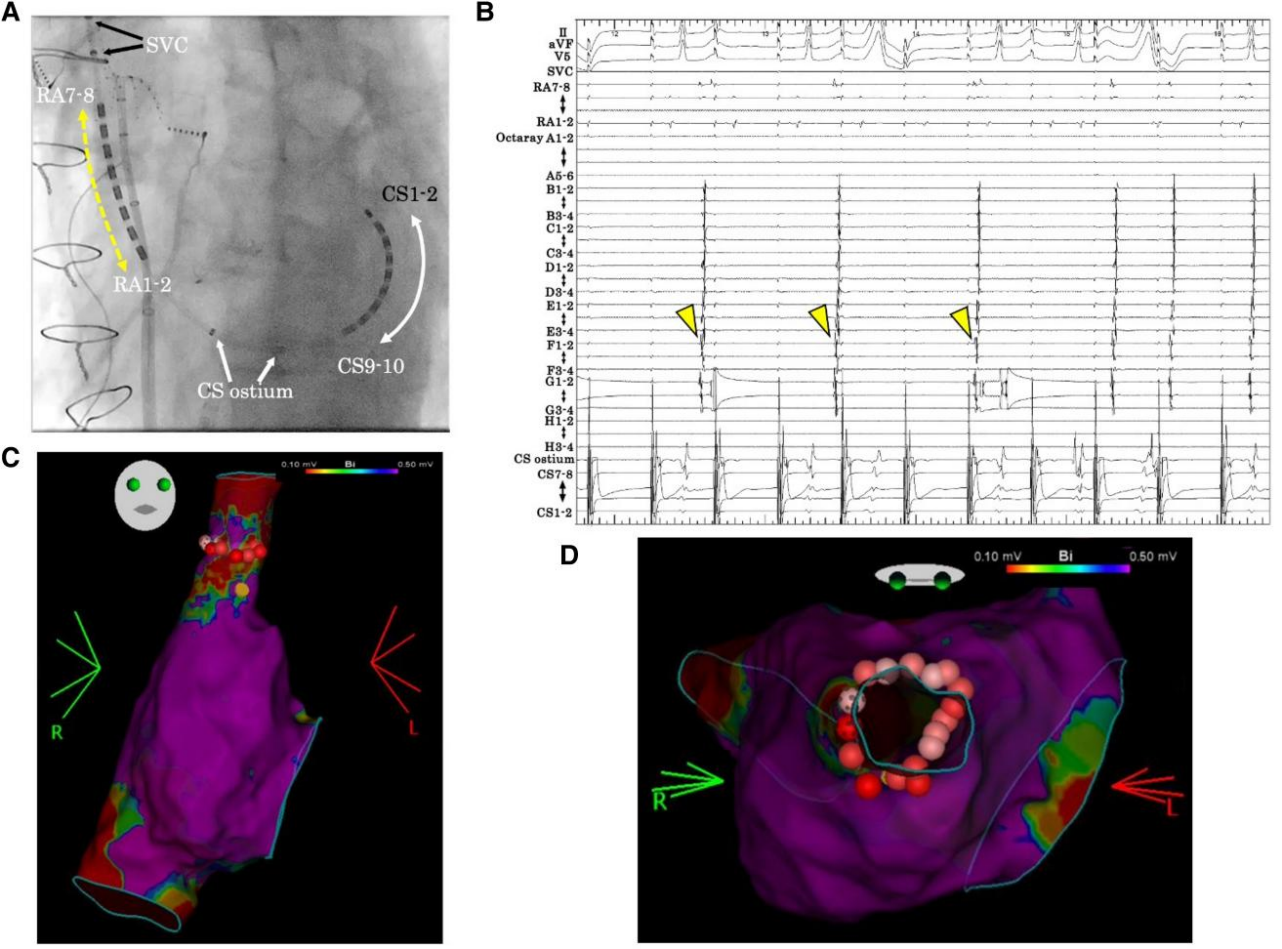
(*A*) Fluoroscopic images showing the position of an A BeeAT catheter in the superior vena cava (black arrow), right atrium (yellow arrow), and coronary sinus (white arrow). The Octaray catheter is positioned in the superior vena cava. (*B*) Intracardiac electrograms after adenosine triphosphate administration. During constant pacing from coronary sinus 5–6, ectopic activity originating from the superior vena cava was observed (yellow arrow). (*C* and *D*) Right atrial activation map after pulmonary vein isolation, merged with left atrial computed tomography image, demonstrating dormant conduction. Superior vena cava isolation was performed using thermal ablation (red tag). High-power, short-duration applications (dotted red tags) were delivered selectively to avoid phrenic nerve injury. Circumferential lesions were created under guidance from three-dimensional electroanatomic mapping, which delineated the electrophysiological position of the sinus node.
